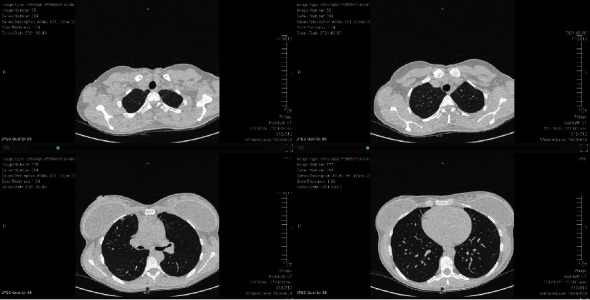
Resolution of disease seen on serial axial cuts of noncontrast chest CT six months after initial presentation.
